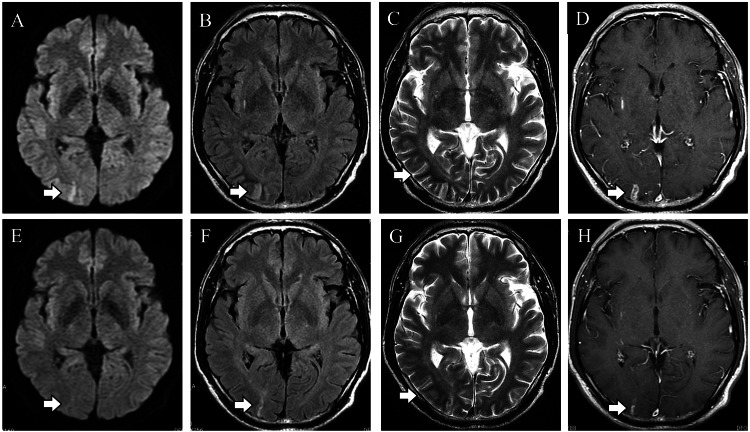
Follow-up brain magnetic resonance imaging. MRI on admission (A-D) and follow-ups (E-H). Hyperintensity lesion in diffusion-weighted imaging and fluid-attenuated inversion recovery improved. Twenty days after admission, T2 low-signal lesions disappeared, and gadolinium-enhanced lesions shrank (E-H, arrows).
